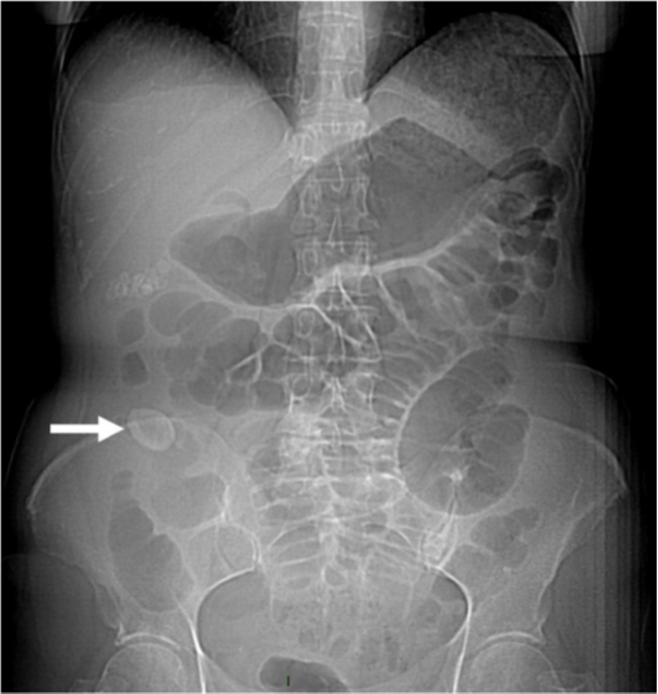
Plain abdominal X-ray showing an obstruction and dilated intestinal loops. Arrow indicates gallstone or possibly the apricot shell.
